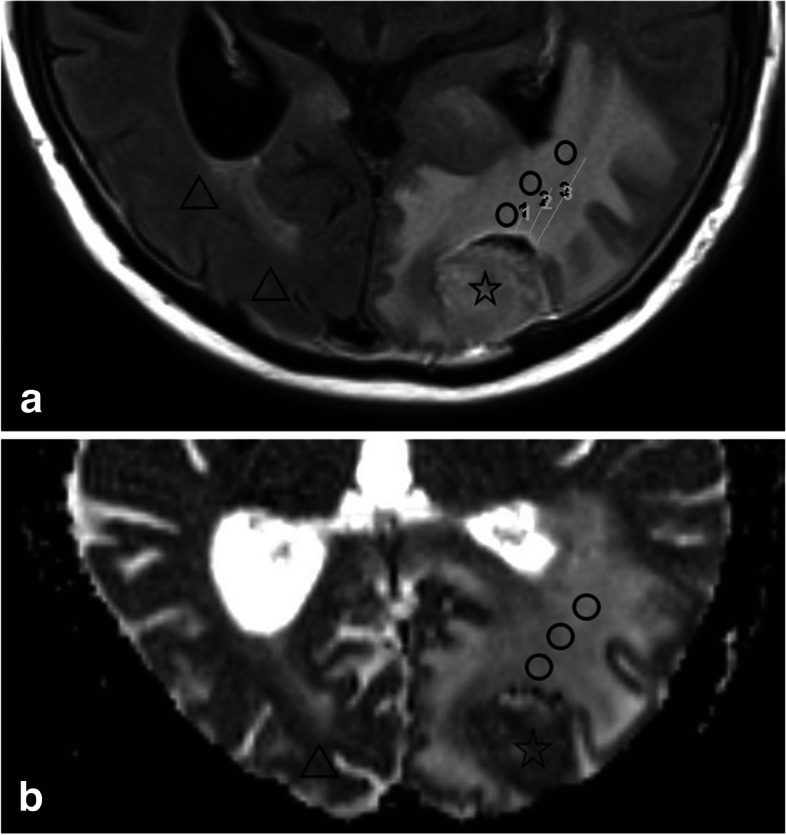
Example of two measurements of the tumor region and edema of multiple brain metastases (a) – T2/-FLAIR-weighted; (b) – ADC map). STAR—measured tumor region; ADC region, TRIANGLE –reference values of the contralateral healthy white matter; CIRCLES – edema in (1) 5 mm, (2) 10 mm and (3) 20 mm distance to tumor border. FLAIR – Fluid attenuated inversion recovery; ADC – apparent diffusion coefficient
